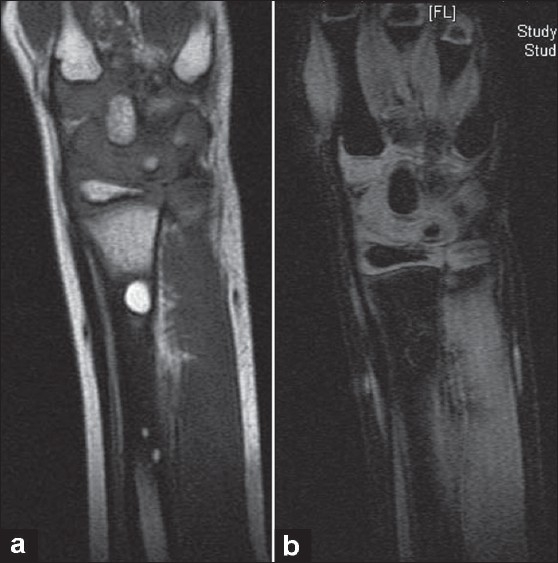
T1WI of MRI (a) at three months follow-up shows area of increased signal with a density similar to that of fat adjacent to the fracture and corresponding to the area of the cystic lesion. Two other coincidental areas of increased signal of unknown significance seen in proximal radial shaft. Fat suppressed image (b) confirming fatty composition of the cyst.
